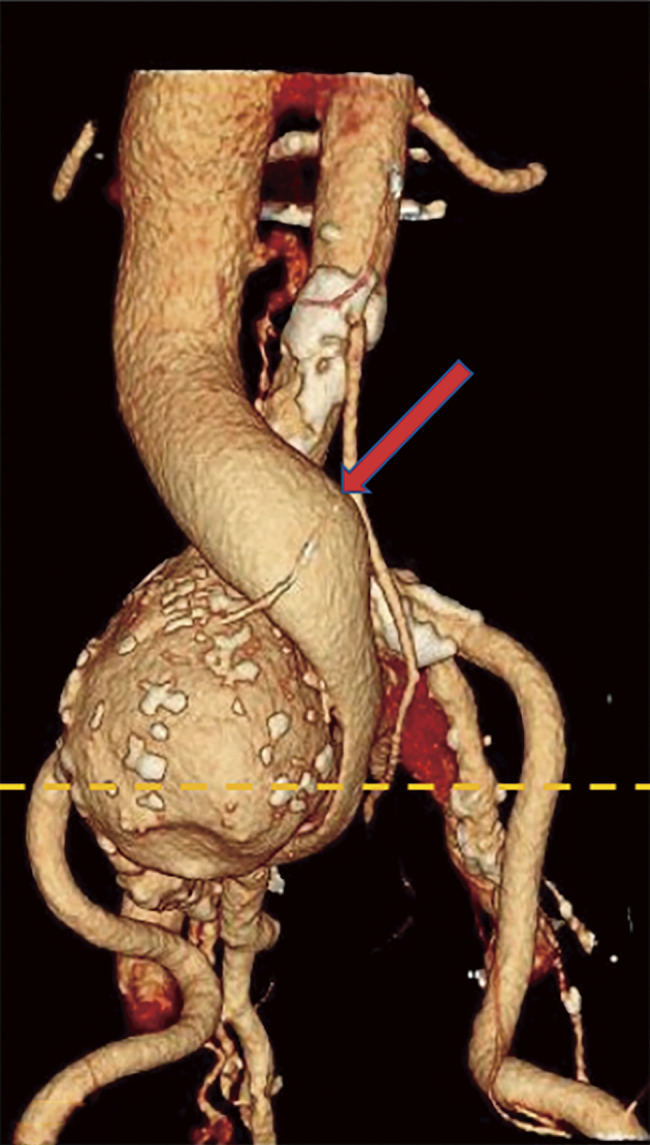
A three-dimensional constructed image, created using contrast-enhanced computed tomography is shown. The yellow dotted line in this image corresponds to the cross-section shown in Fig. 2. The IVC runs anterior to the right CIAA and is dilated on the proximal side (red arrow). CIAA, common iliac artery aneurysm; IVC, inferior vena cava
